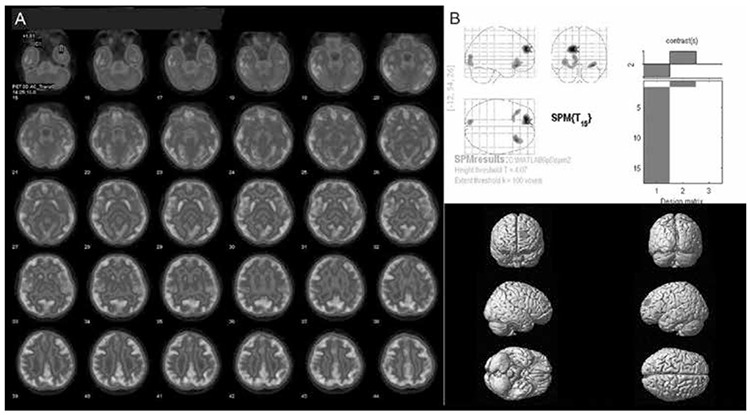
Interictal fluorine-18 fluorodeoxyglucose positron emission tomography study of a patient with epilepsy with cortical dysplasia detected on magnetic resonance imaging in the left frontal and occipital lobes. The axial views (A) show left frontal and occipital cortical hypometabolism visually that were also evident on the quantitative analysis by statistical parametric mapping (B)
